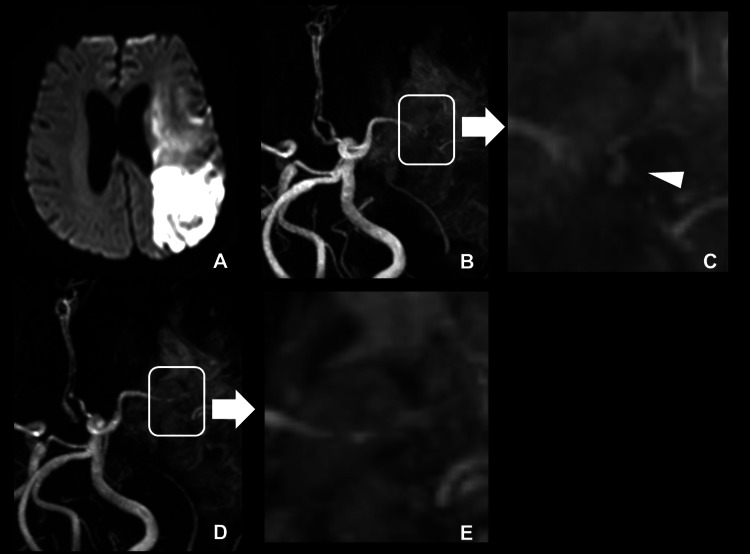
Follow-up MRI and MRA findings on day 18 and day 28 (A) Follow-up MRI performed on day 18 revealed progression of cerebral infarction in the left MCA territory. (B) MRA on the same day showed severe vasospasm and a suspected pseudoaneurysm in the left MCA (white box). (C) Enlarged view of the area marked by the white box in panel B, demonstrating the suspected pseudoaneurysm (white arrowhead). (D) Follow-up MRA on day 28 demonstrated disappearance of the pseudoaneurysm (white box). (E) Enlarged view of the area marked by the white box in panel D, demonstrating no residual pseudoaneurysm. MRI, Magnetic resonance imaging; MRA, Magnetic resonance angiography; MCA, Middle cerebral artery
